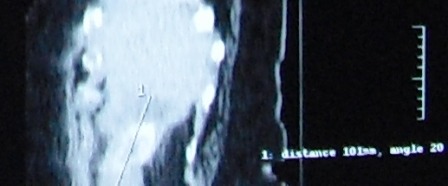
Abdominal CT showing the calculus and the subhepatic abscess (enhanced image)
